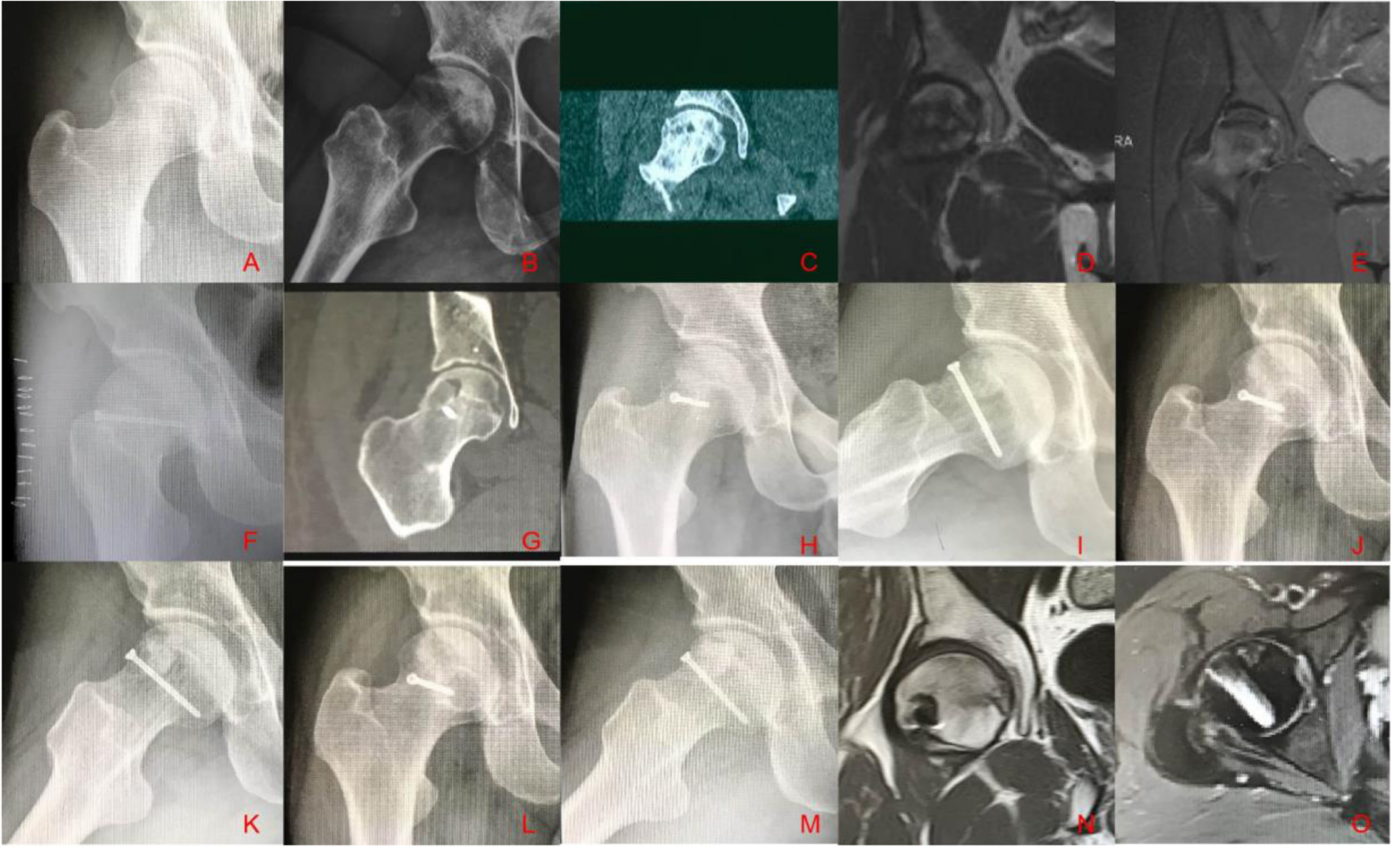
A 32-year-old man with osteonecrosis of the femoral head was treated with our modified trapdoor procedure. Anterior–posterior X-ray (a) and frog-position X-ray (b) show femoral head necrosis with segmental collapse. Coronal CT confirmed ONFH with collapse (c). Coronal T1 (d) and STIR (e) showed ONFH with edema. Postoperative radiography (f) showed necrotic bone that had been curetted and replaced with tricortical iliac block graft. Coronal CT (g) showed that necrotic bone had been curetted and replaced with a tricortical iliac block graft. The graft was in accordance with the contour of the femoral head. Anterior–posterior X-ray (h) and frog-position X-ray (i) obtained 1 year postoperatively show that the graft had healed to the host bone without collapse. Anterior–posterior X-ray (j) and frog-position X-ray (k) obtained 4 years postoperatively show that the graft had healed to the host bone, without collapse. Anterior–posterior X-ray (l) and frog-position X-ray (m) obtained 8 years postoperatively show that the contour of the femoral head was intact without collapse. Coronal T1 MR images (n) obtained 4 years postoperatively showed that the contour of the femoral head was intact, with the replacement of the necrotic bone by a viable bone, and normal cartilage at the femoral head. Axial STIR MR images (o) obtained 4 years postoperatively show that the contour of the femoral head remained intact; a portion of the necrotic bone has been replaced with a viable bone
